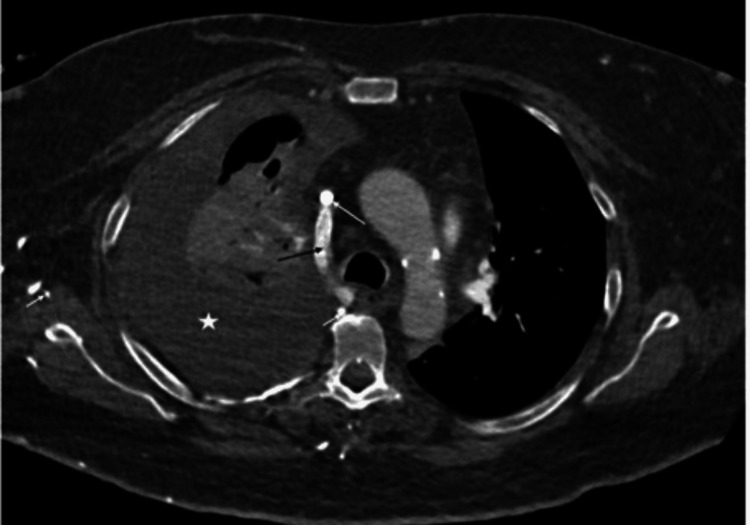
Axial post-contrast CT study of the thorax Axial post-contrast CT image of the thorax after intravenous injection into the right subclavian vein above, at, and below the level of the azygous vein-superior vena cava (SVC) confluence shows massive right-sided pleural effusion in keeping with chylothorax (white asterisk). A right-sided central venous catheter is noted with non-visualization of an enhancing SVC, suggesting chronic thrombosis (long white arrow).
